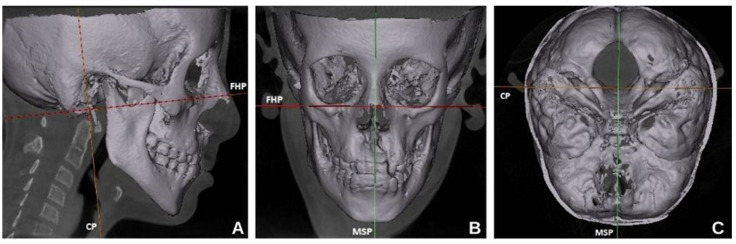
CBCT images with the orthogonal planes. FHP—Frankfurt horizontal plane (A), MSP—midsagittal plane (B), CP—coronal plane (C).
